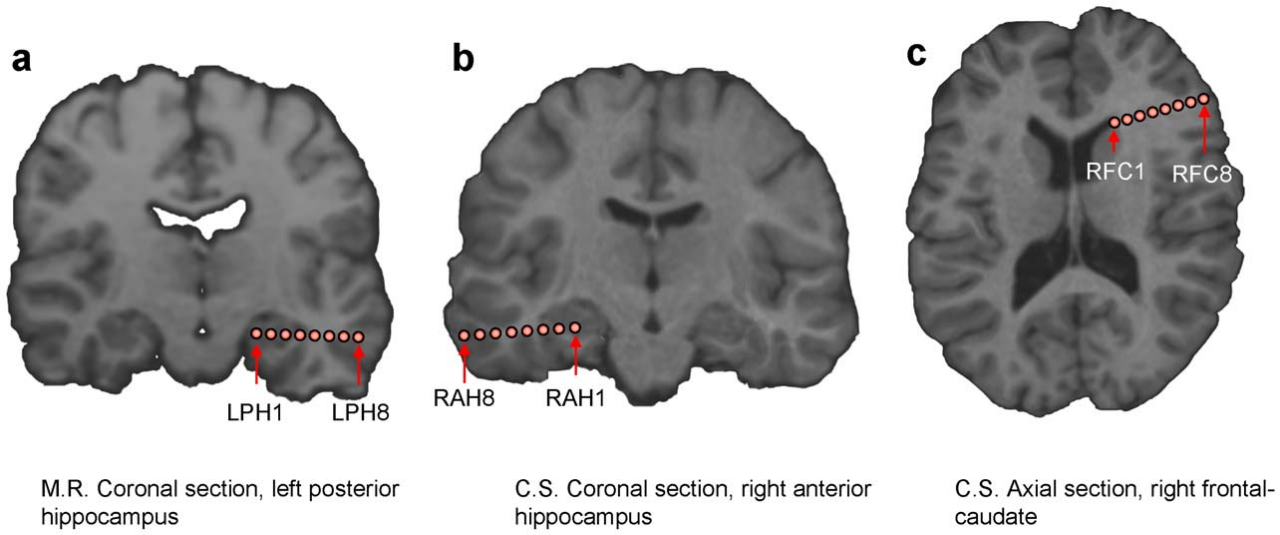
Visualization of the areas most contributing to the classification. (a) Left posterior hippocampus for patient M.R. (b) Right anterior hippocampus and (c) right frontal-caudate region for patient C.S. Electrodes localized by the CT scan were coregistered to the MRI T1-weighted brain volumes.
